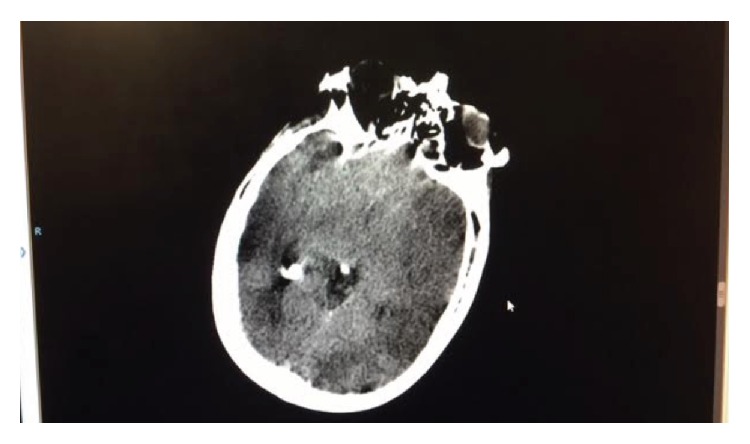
Head computed tomography image without contrast, showing left middle cerebral artery territory infarction.
